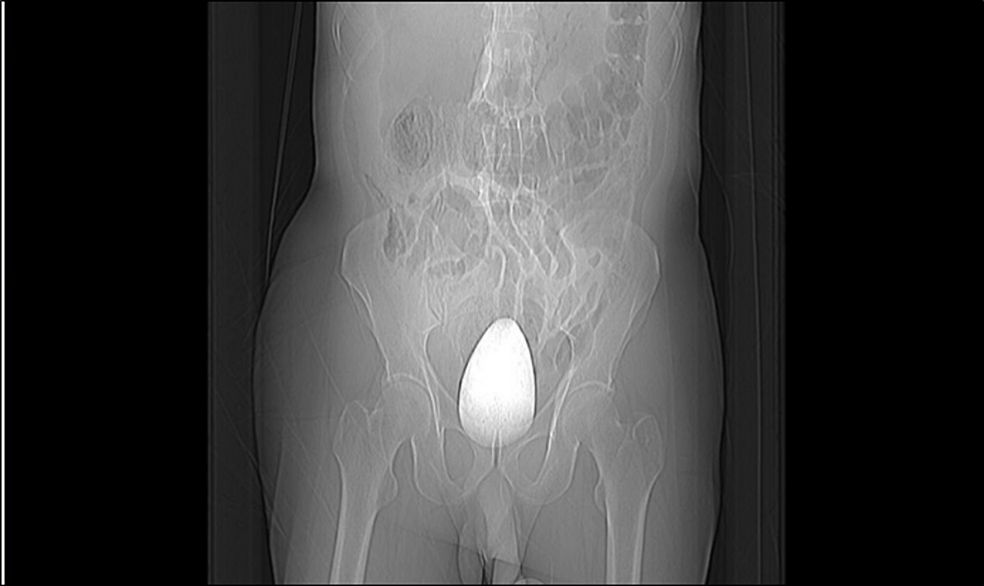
Abdominal x-ray showing the presence of a large foreign body in rectum.
